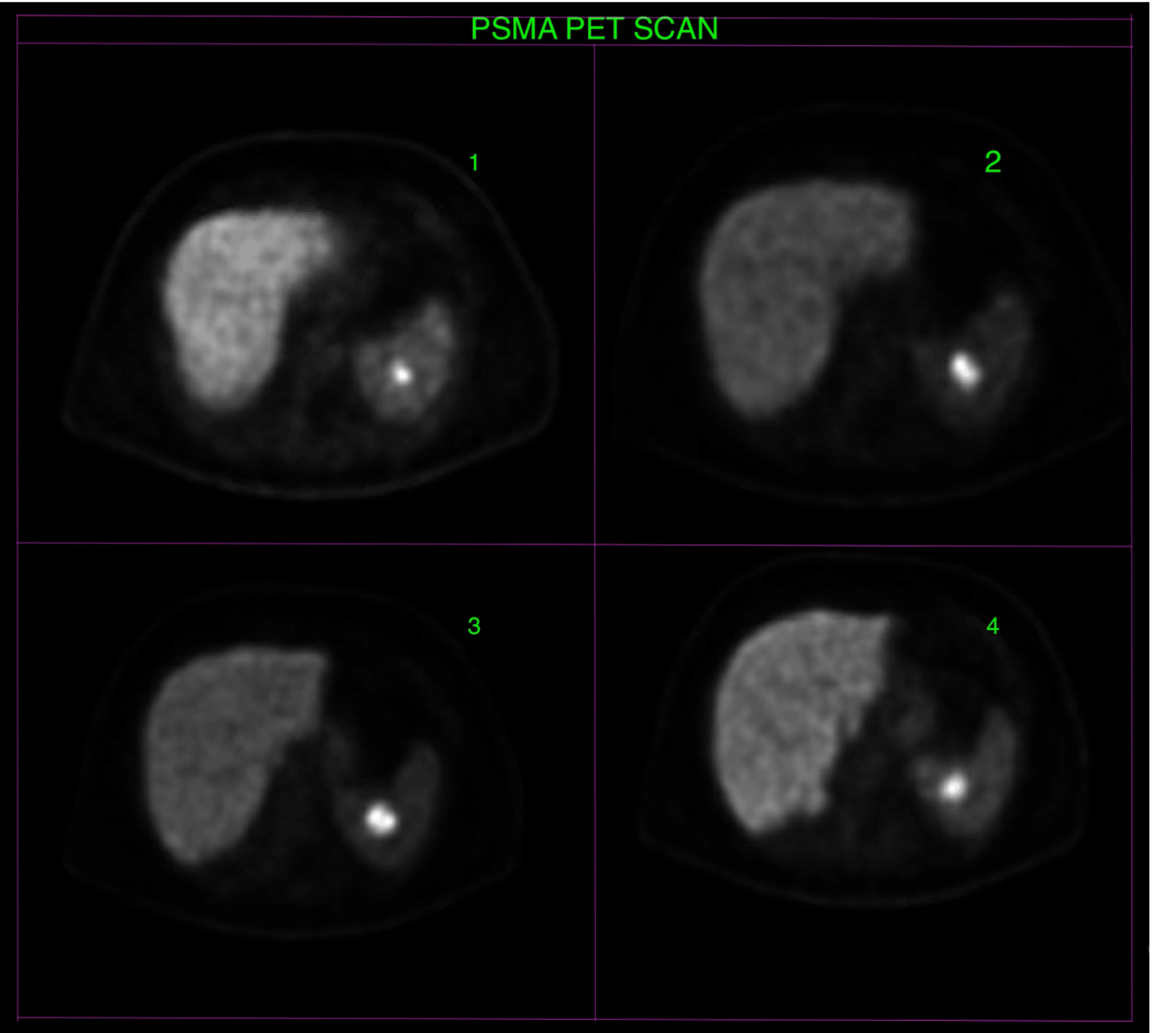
PSMA PET/CT images showing focal intense tracer activity in the spleen. PSMA = prostate-specific membrane antigen; PET/CT = positron emission tomography/computed tomography
